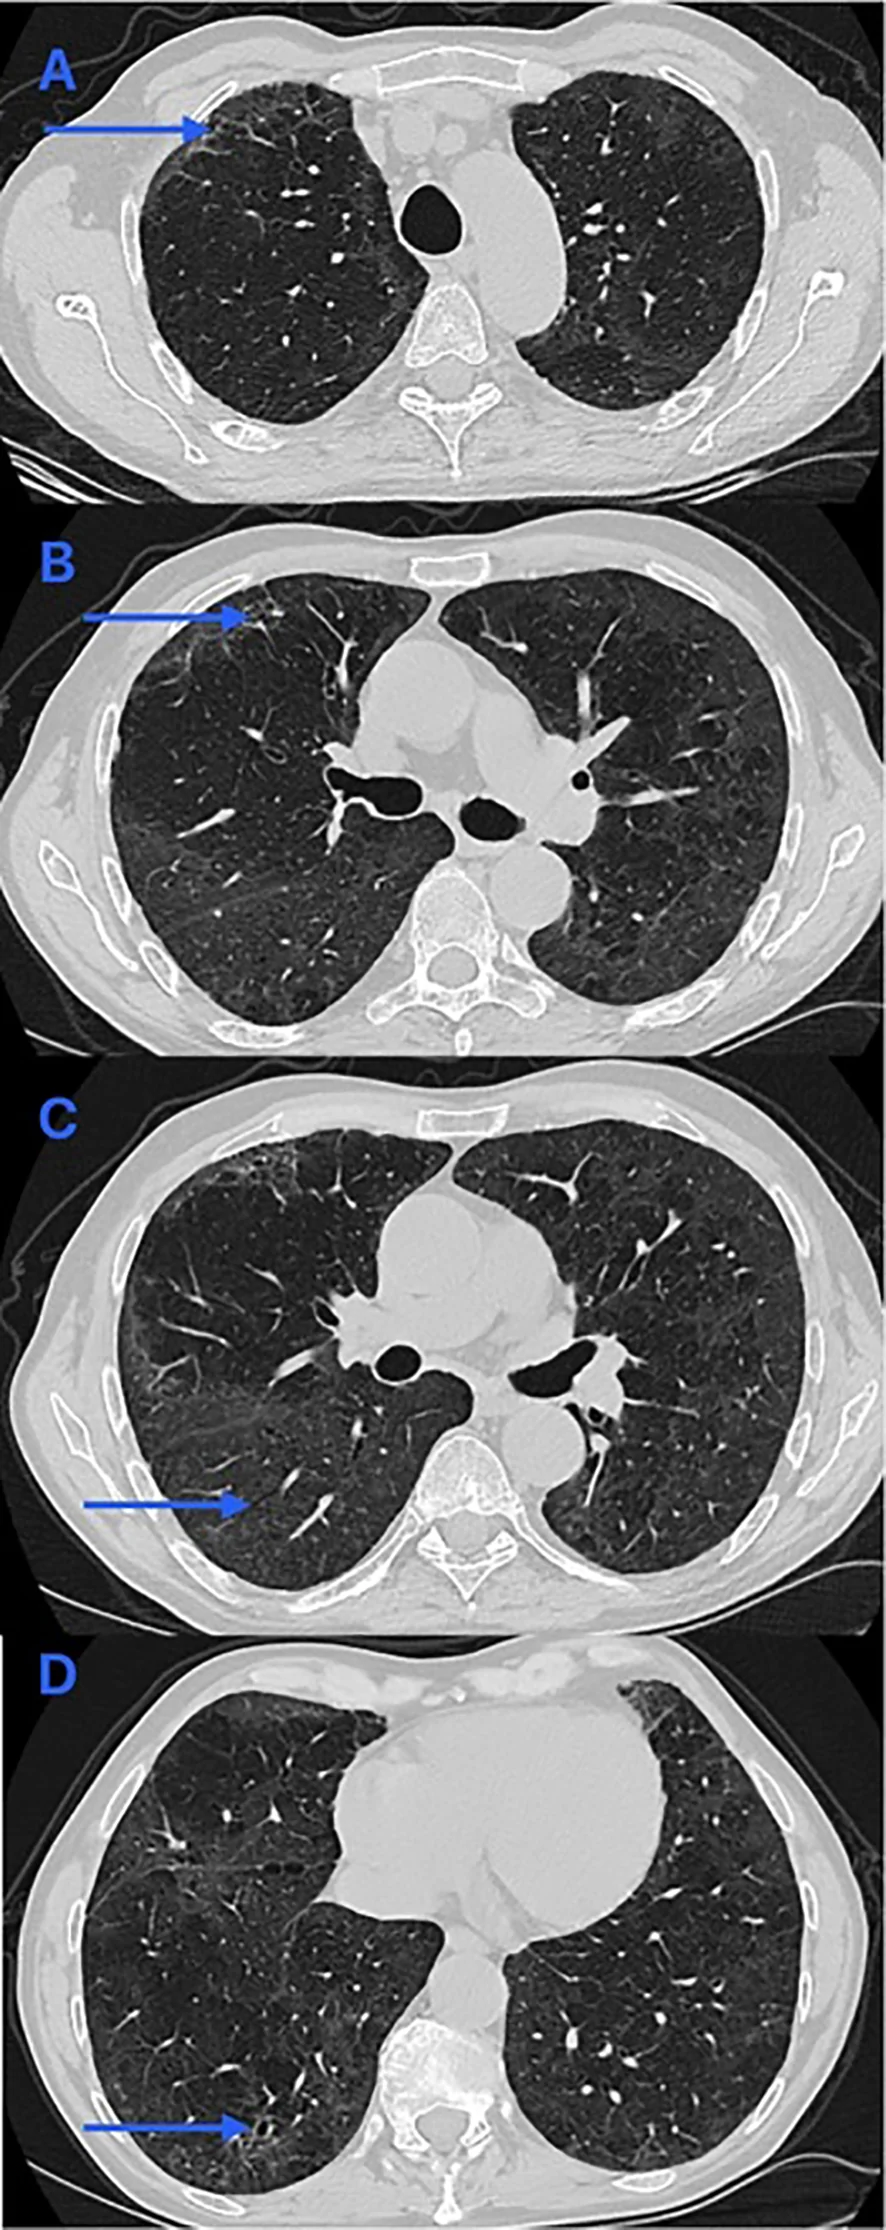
Follow-up chest CT at three weeks demonstrating significant regression of ground-glass opacities with (blue arrows): (A, B) limited honeycombing, consistent with NSIP/UIP overlap. (C, D) residual traction bronchiectasis.
